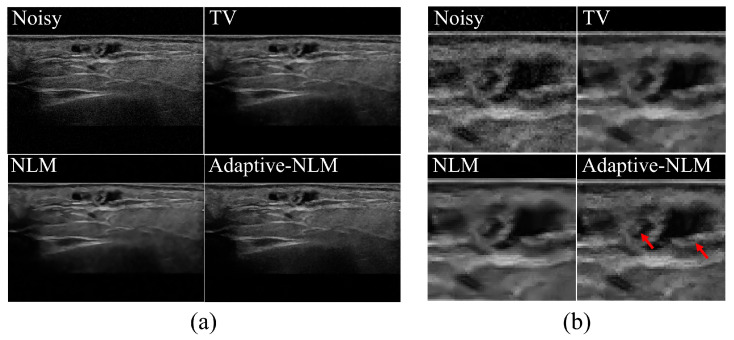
Results of applying the noise reduction methods to the UI after 1 year in the patient with parotid malignant tumors shown in Figure 4. (a) Noisy image from applying conventional TV, the NLM algorithm, and the proposed adaptive NLM algorithm, and (b) UI resulting from enlarging the ROI_A_ area in Figure 3b. The red arrow indicates that the edge can be clearly identified, which is one of the ultrasound imaging findings of malignant tumors.
